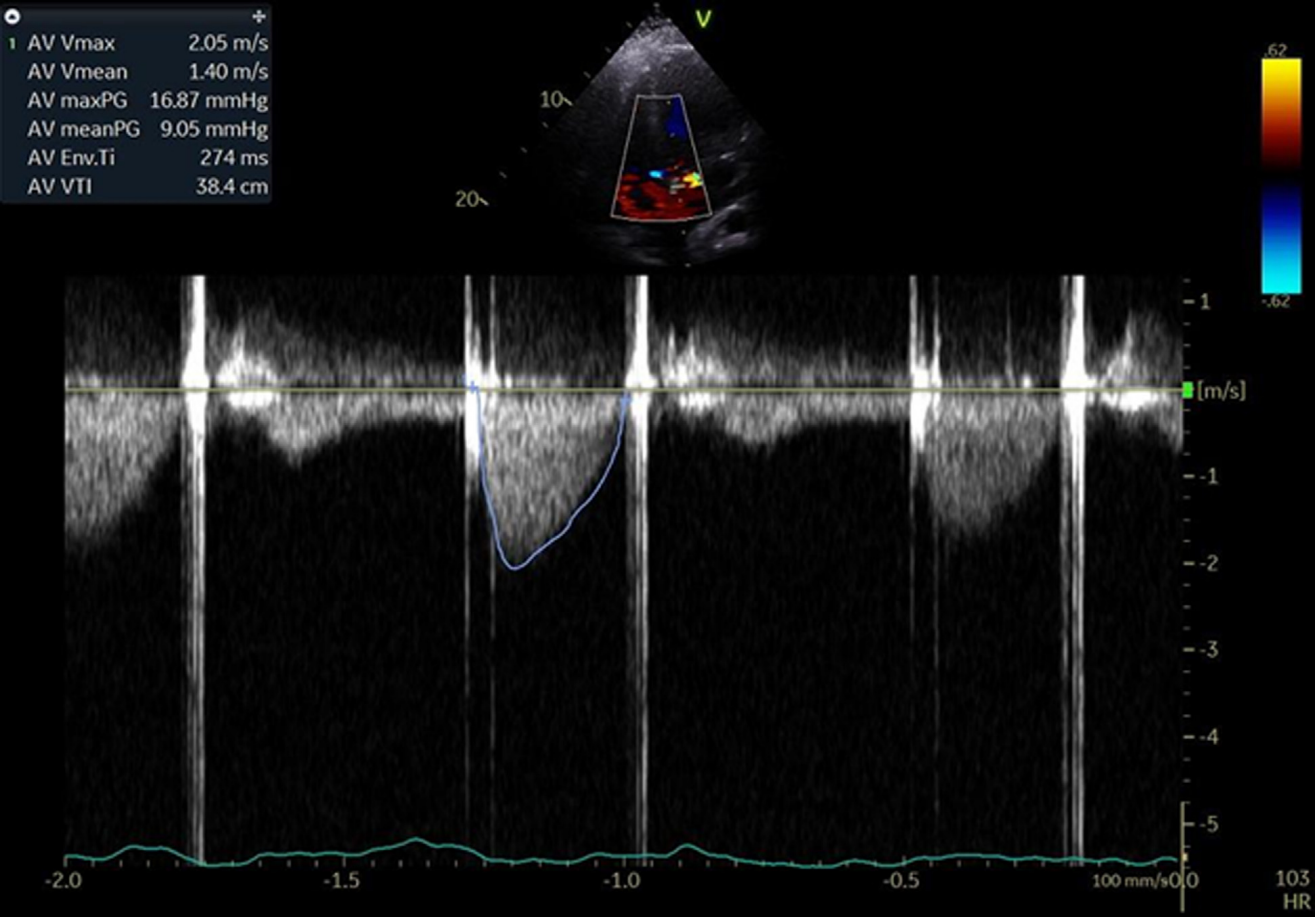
Transthoracic Echocardiography After the Second Cycle of Tissue Plasminogen Activator Transthoracic echocardiography showing a much improved peak and mean transvalvular gradient of 16 mm Hg and 9 mm Hg, respectively.
